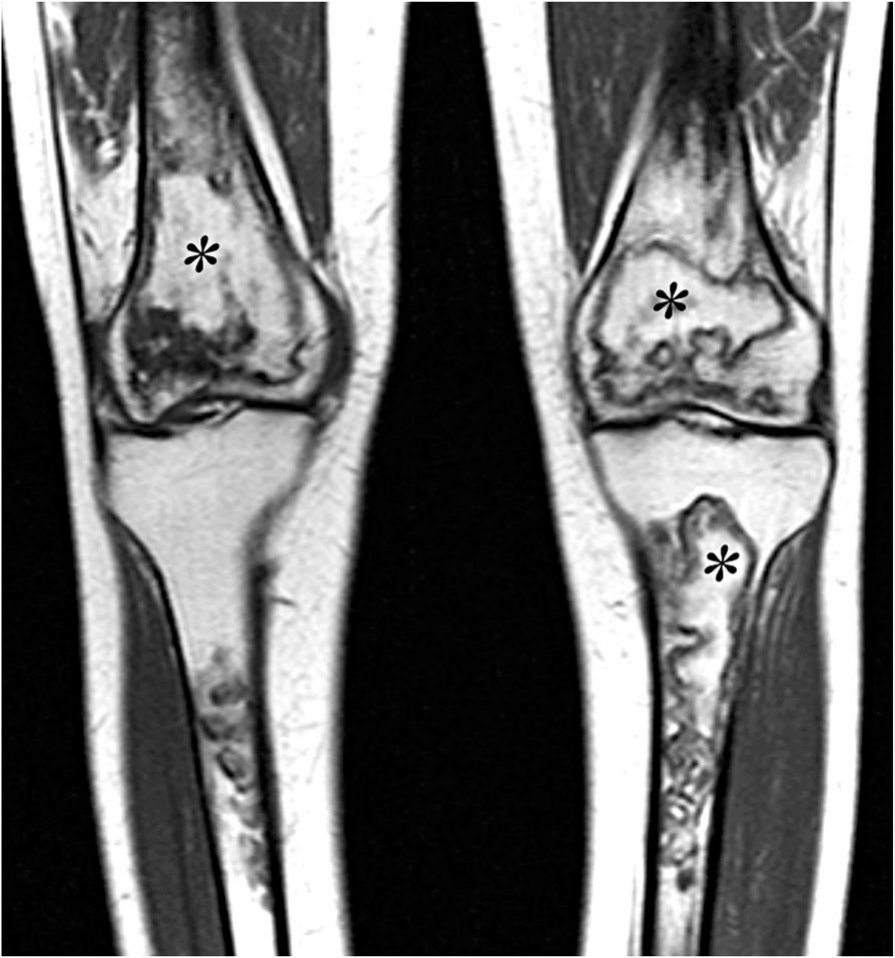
Distribution of osteonecrosis from systemic origin. T1-weighted MR image shows lesions characterized by bone marrow areas surrounded by a peripheral rim (asterisks). These lesions are often bilateral, epiphyseal, and diaphyseal-metaphyseal. The areas within the peripheral rims may show a normal marrow signal, corresponding to mummified but not degraded fat
